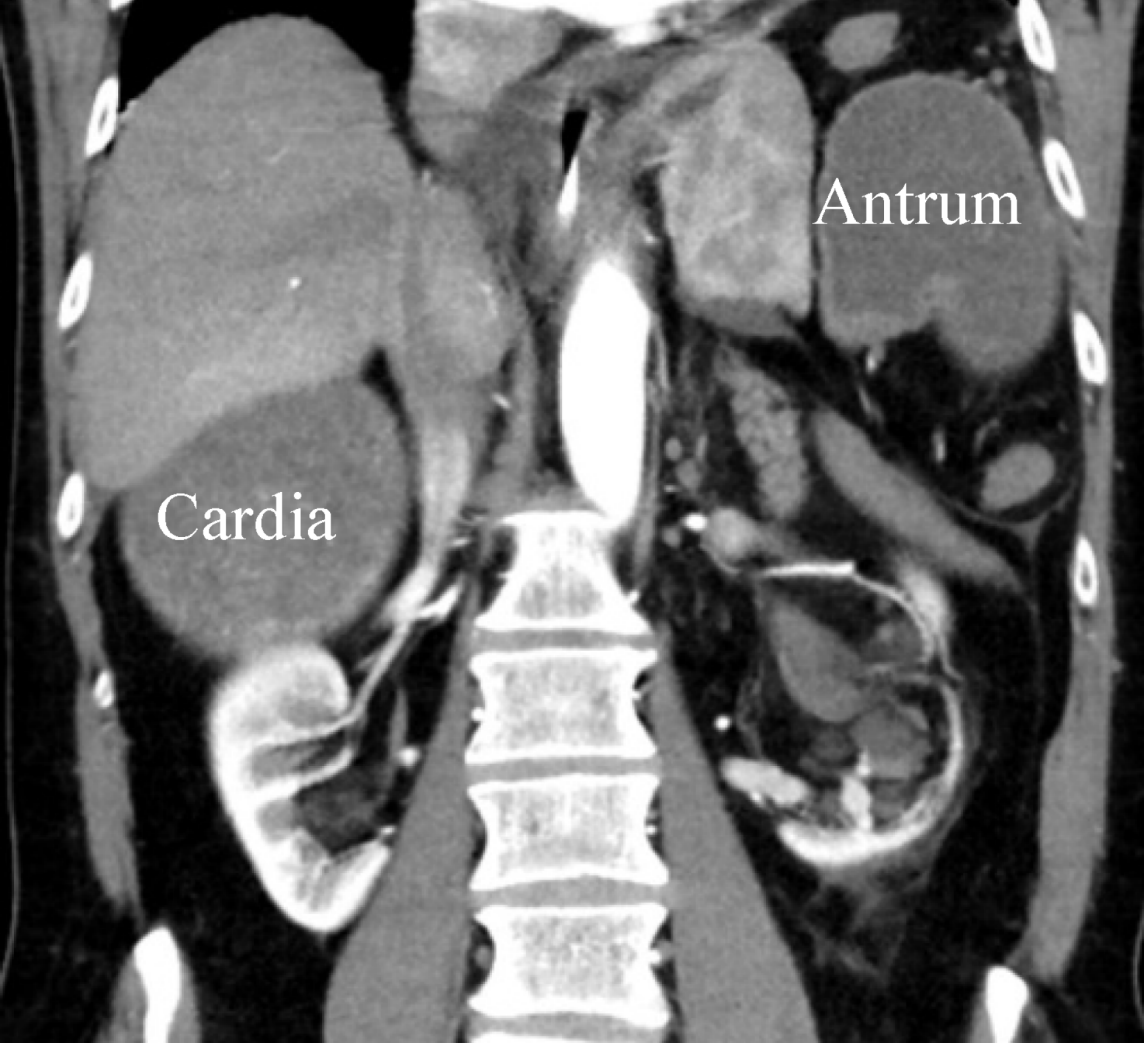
CT scan: dislocation of the cardia to the right lower epigastrium and the antrum to the upper left abdomen
